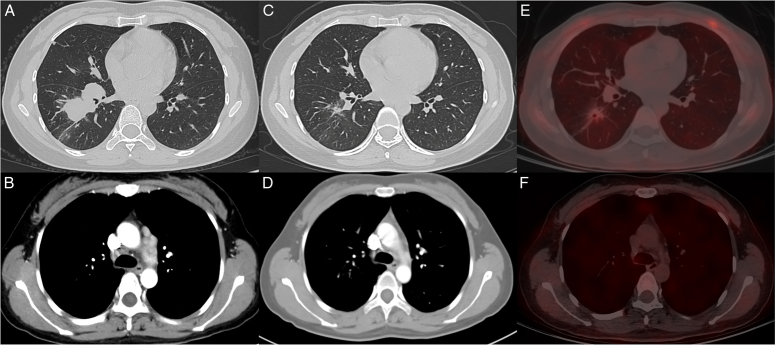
A case about conversion surgery for initially unresectable NSCLC. A 35-year-old female presented with a mass in the right lower lobe of the lung (A), accompanied by enlarged contralateral mediastinal lymph nodes (B). Preoperative biopsy confirmed the diagnosis of lung adenocarcinoma and mediastinal lymph node metastasis (cT3N3M0, stage IIIC), with an epidermal growth factor receptor exon 19 deletion. Initially, the patient received two months of treatment with almonertinib. The tumor responded well to almonertinib, with significant shrinkage of the primary lesion (C) in the right lower lobe and the disappearance of enlarged contralateral mediastinal lymph nodes (D). In the further assessment with PET/CT, the SUVmax value of the primary lesion was 1.91 (E), with no abnormal uptake in the hilar and mediastinal lymph nodes (F). Subsequently, the patient underwent right lower lobe resection and systematic lymph node dissection, with postoperative pathological staging revealing ypT1cN2M0, stage IIIA. Postoperatively, the patient continued to receive almonertinib therapy. Up to now, the patient has been followed up for 1 year and remains alive with no recurrence. NSCLC, nonsmall cell lung cancer; PET/CT, positron emission tomography/computed tomography; SUV, standardized uptake value.
